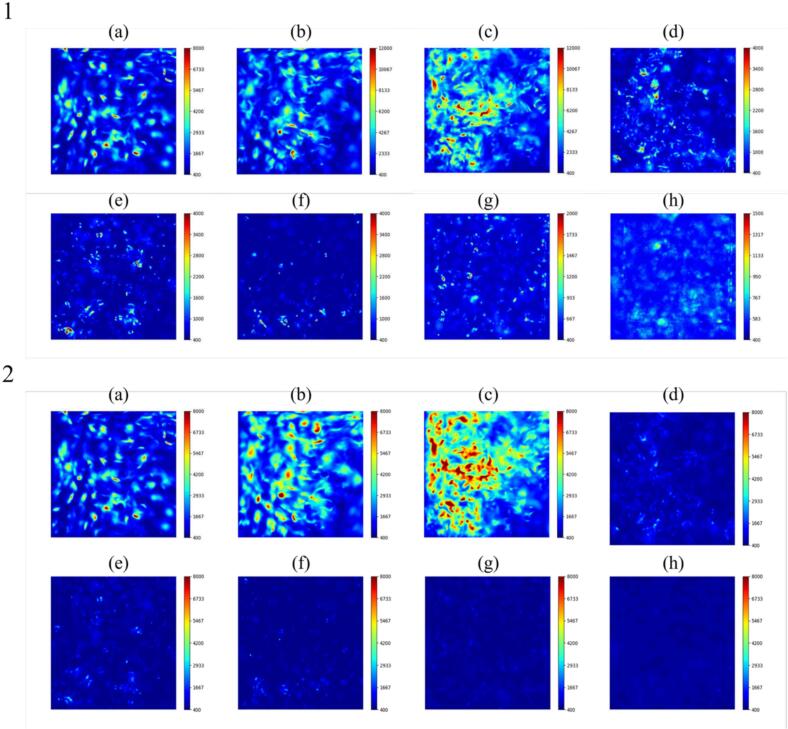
Distribution of sono-chemiluminescence (SCL) intensity and its reaction zone in the ultrasonic bath taken from a window frame (50 mm × 50 mm) with the ultrasonic power of (a) 18 W, (b) 33 W, (c) 43 W, (d) 49 W, (e) 69 W, (f) 84 W, (g) 117 W, and (h) 136 W. The color scales represent the intensity of SCL. 1. The color scale is varied for its visibility and 2. The color scale is same for all powers.
